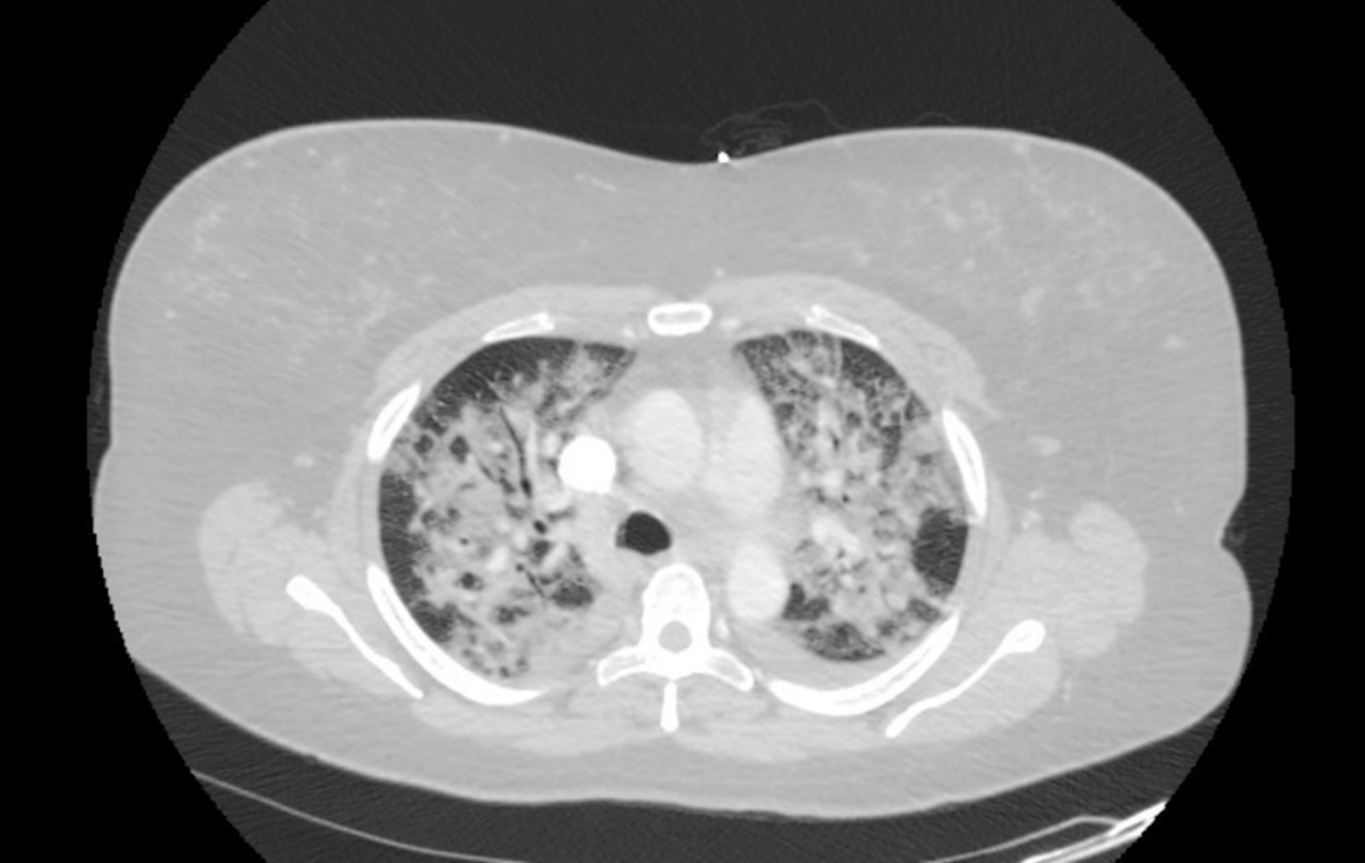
CTA chest showing bilateral alveolar opacities. CTA: Computed tomography angiography
